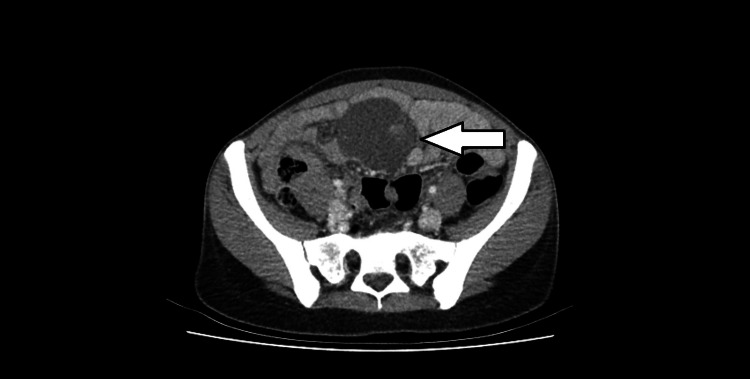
An axial abdominal CT scan image showing lobulated, fluid-attenuating mass. Mass closely abuts on small bowel loops.
